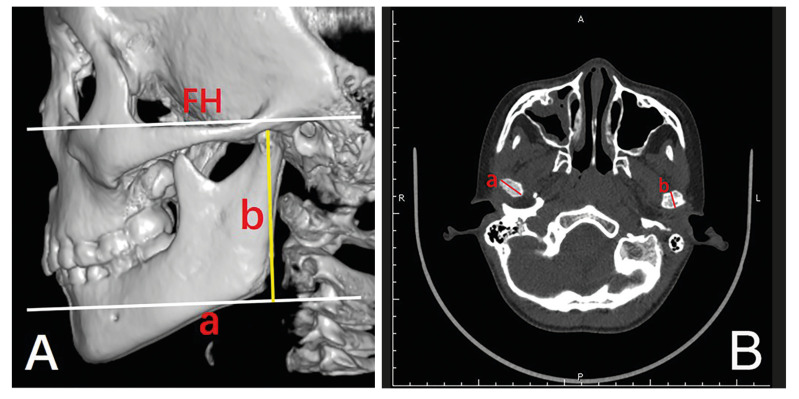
A) The measurement methods of MRH in the spiral multi-slice CT image: a line parallel to the Frankfort horizontal (FH) plane through the point of the ante gonial notch; b, ramus height: distance from the most superior end of the condyle to line a; B) The measurement methods of APD and MLD of the condylar process based on axial plane image: a mediolateral diameter of the condylar process; b, anteroposterior diameter of the condylar process).
